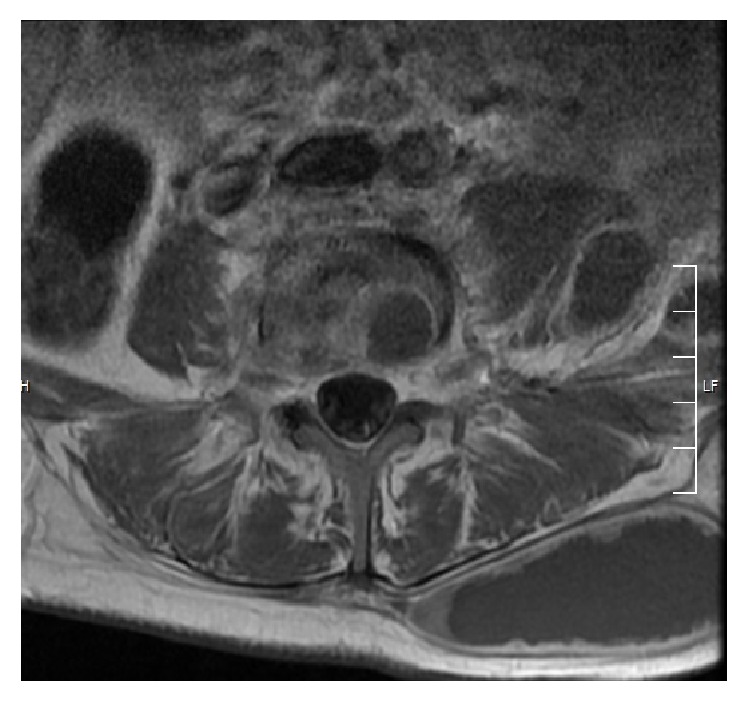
Axial view of MRI lumbar spine. T1 image with contrast depicting edema and erosive changes within vertebral body consistent with diskitis and osteomyelitis. Also figure demonstrates abscess extending into ventral epidural space.
